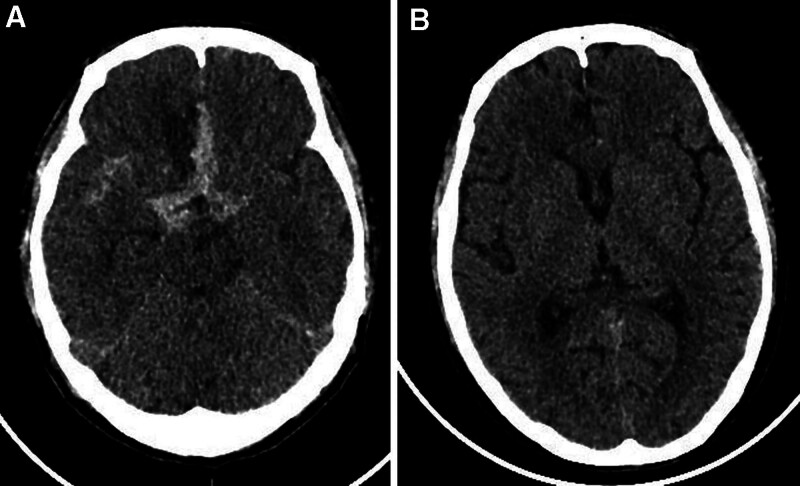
(A) Head CT findings at day 0: a high-density shadow in the cistern of the cerebral longitudinal fissure, cistern of the lateral fossa on both sides, the ambient cistern, and partial cerebral sulci on both sides suggests SAH. (B) Head CT findings at month 2 post-aneurysm treatment. Postoperative changes in the aneurysm can be observed, and the previous high-density shadow in the cistern of the cerebral longitudinal fissure, the right lateral fissure pool, and some brain grooves on both sides have been absorbed. CT = computed tomography, SAH = subarachnoid hemorrhage.
